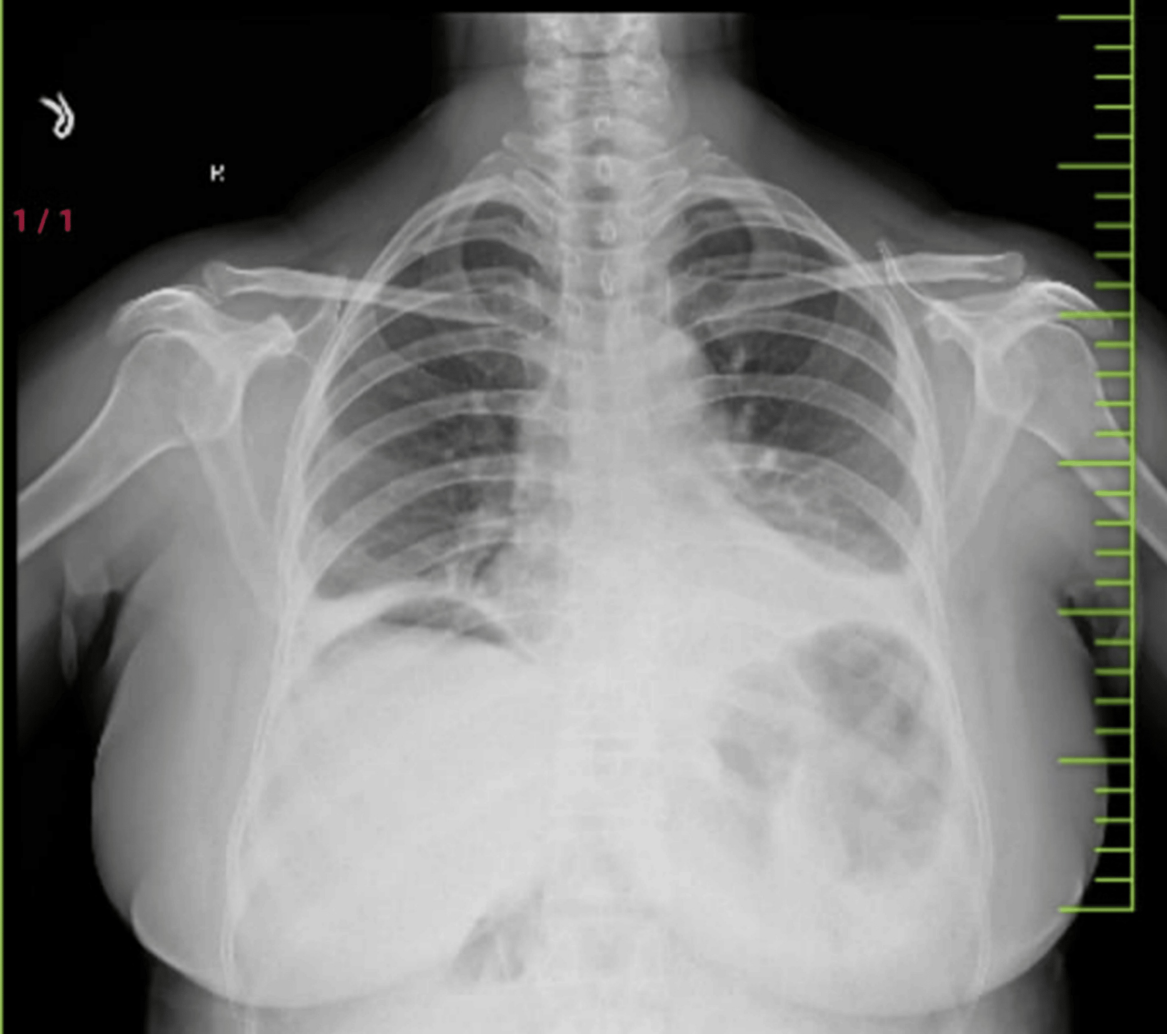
X-ray erect abdomen of a 53-year-old female patient showing gas under the diaphragm on the right side.
